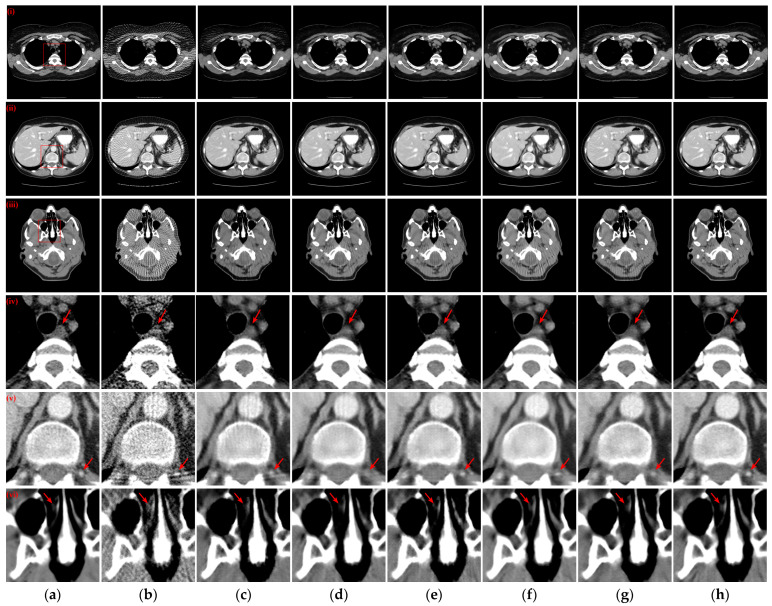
The SVCT reconstruction results from different methods with 128 projection views. (a) The reference image; (b) FBP; (c) MWNet; (d) DuDoTrans; (e) DDPTransformer; (f) MIST; (g) RegFormer; (h) Ours. (i)–(iii) are the chest CT, abdominal CT, and head CT, respectively, and (iv)–(vi) correspond to the enlarged ROIs marked by the red boxes in the reference images above. The display window is [−160, 240] HU.
